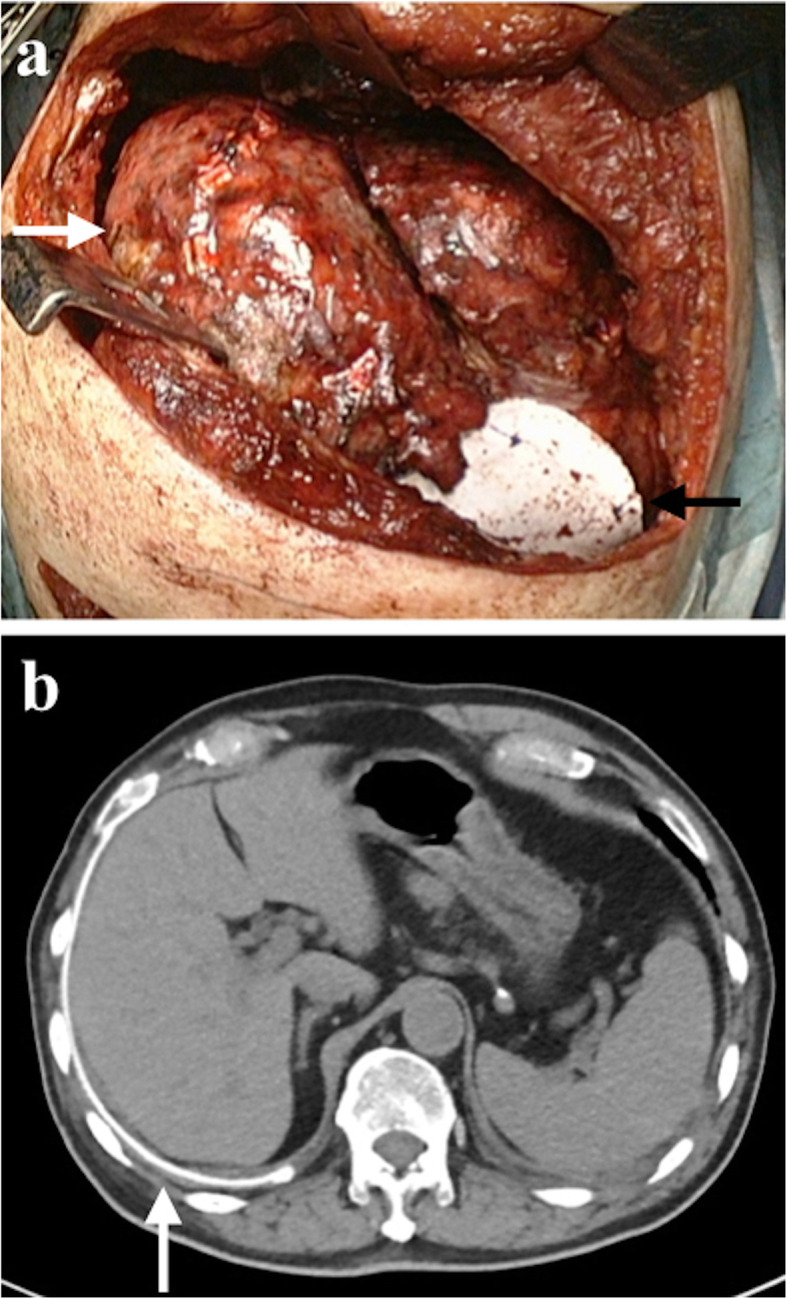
Case 4. A 62-year-old man with a right malignant pleural mesothelioma. (a) Intraoperative view. Right lung after decortication is seen in the left thoracic cavity (white arrow). Part of the right diaphragm was resected, and the defect was repaired with GORE DUALMESH (black arrow). (b) Computed tomography 1 month after surgery. The mesh remains in place without any sign of complication (white arrow)
